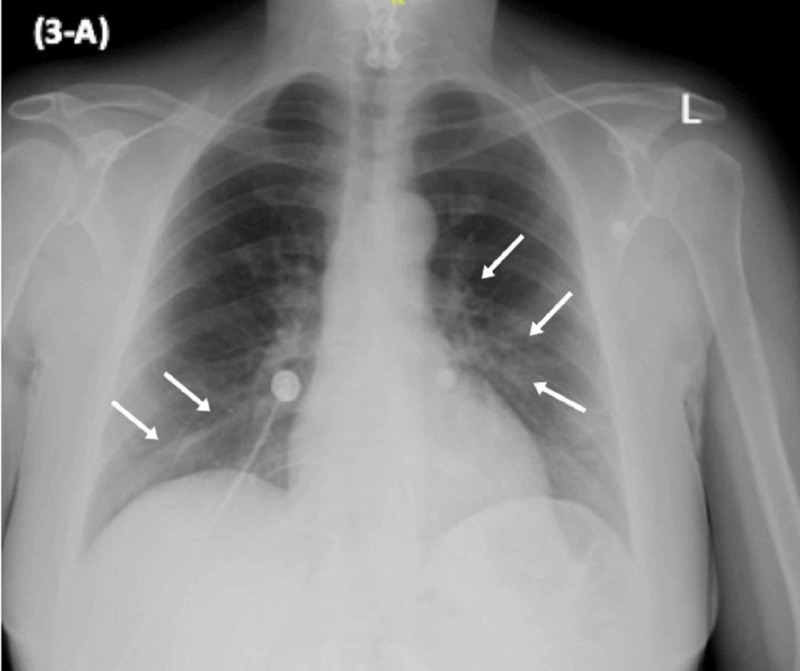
CXR showing bilateral patchy areas of multifocal infiltrate. CXR, Chest X-ray
